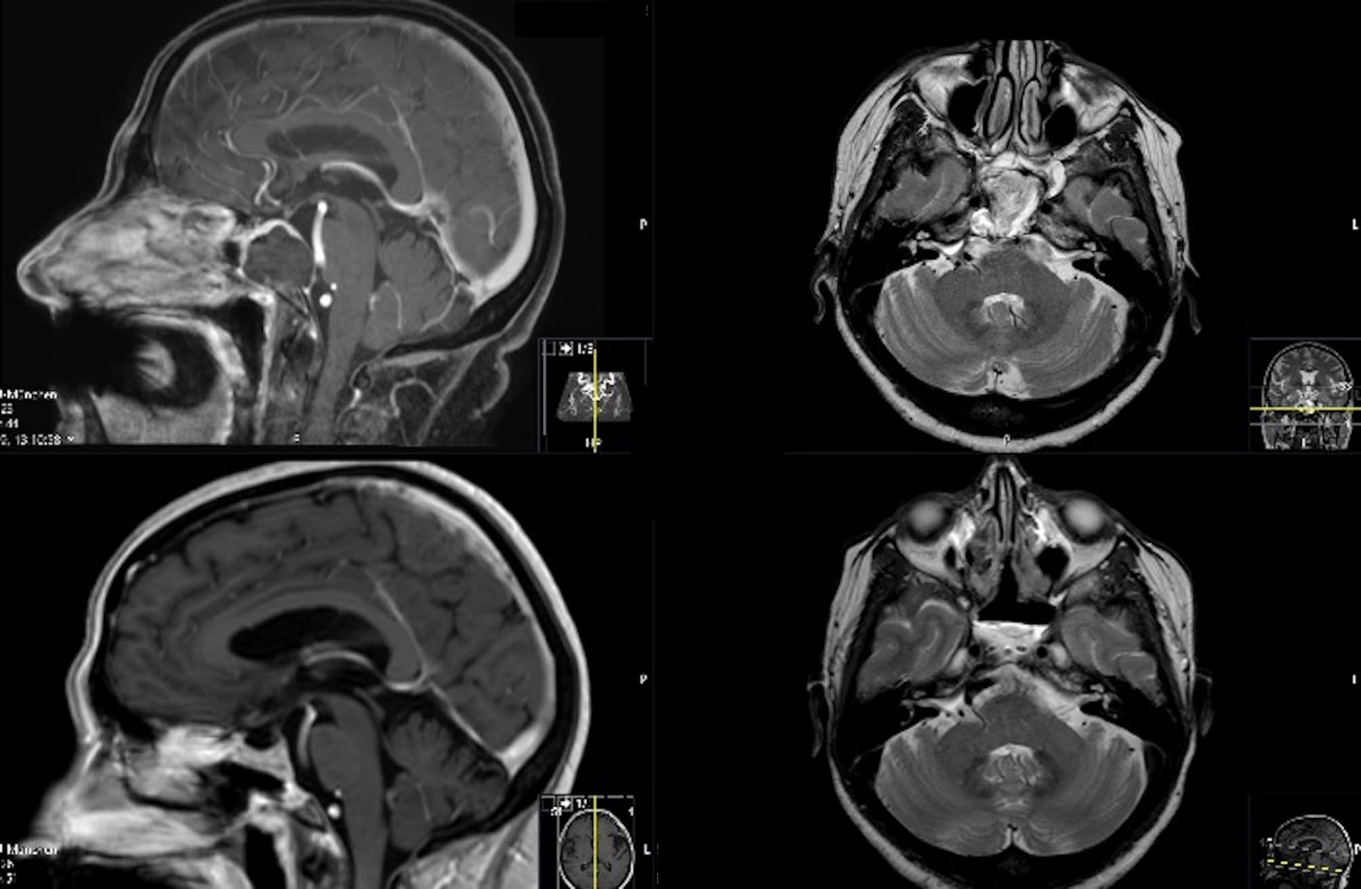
Preoperative T1 with contrast enhancement and T2-weighted MRI scan of a 60-year-old female patient suffering from a clival chordoma (upper images), with postoperative MRI revealing a gross total tumor resection after the transnasal approach.
